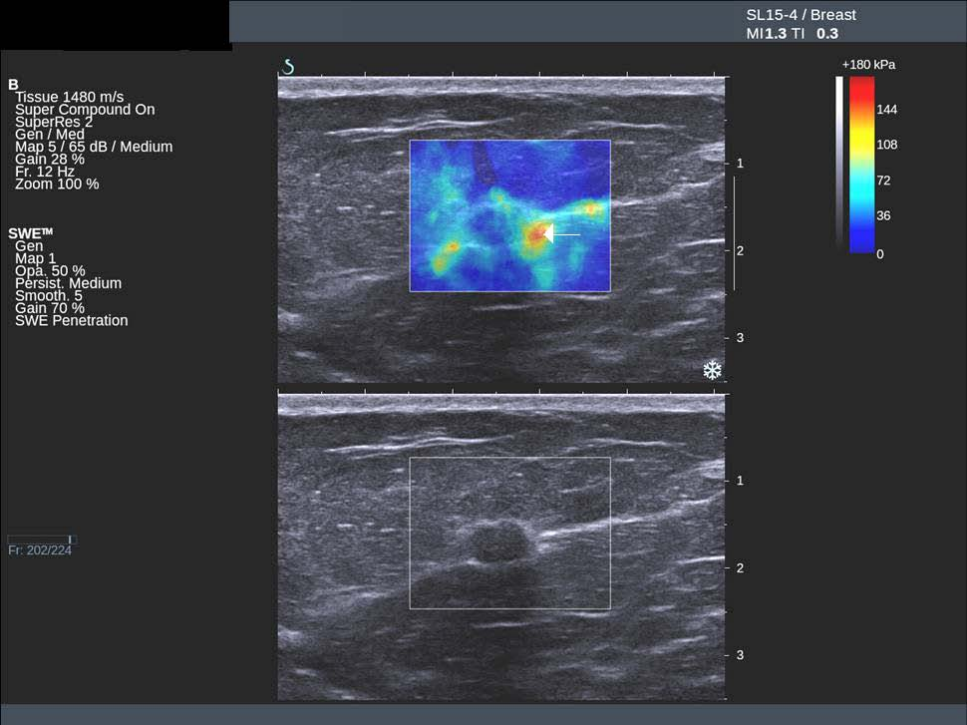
Ultrasound and elastography images of an invasive cancer. Ultrasound and elastography images showing an invasive cancer with benign greyscale features but suspicious peri-tumoural stiffness on the elastography image (arrow).
